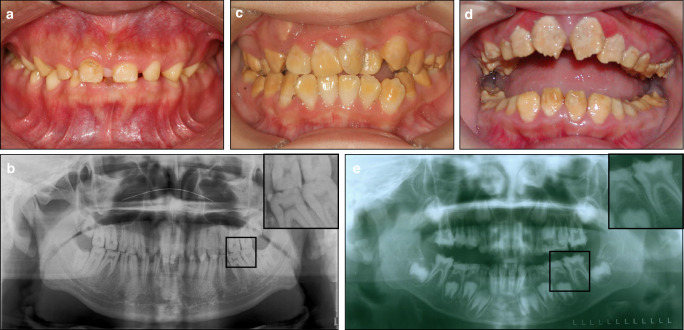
Amelogenesis imperfecta (AI). a, b Hypoplastic AI in a 26-year-old male. a The patient exhibits a generalized reduction in enamel thickness, leading to widely spaced teeth and a lack of proximal contact. b The radiograph reveals insufficient enamel thickness. c Hypomaturation AI in a 13-year-old female. The patient shows uneven yellow discoloration of the enamel. d, e Hypocalcified AI in a 9-year-old male. d The patient presents with a rough enamel surface covered with scales. e The radiograph shows reduced contrast between the enamel and dentin
